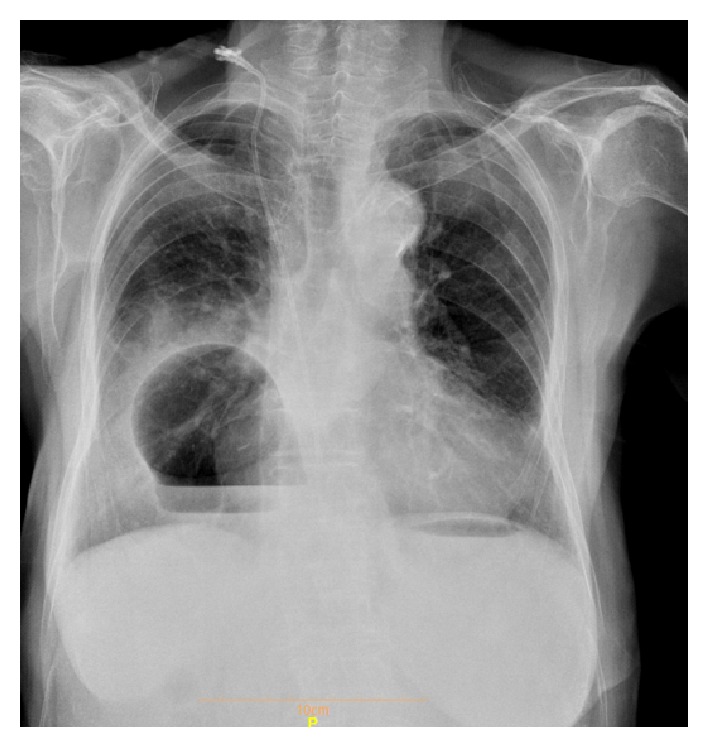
Chest X-ray. Mass with air-fluid level in right hemithorax.
